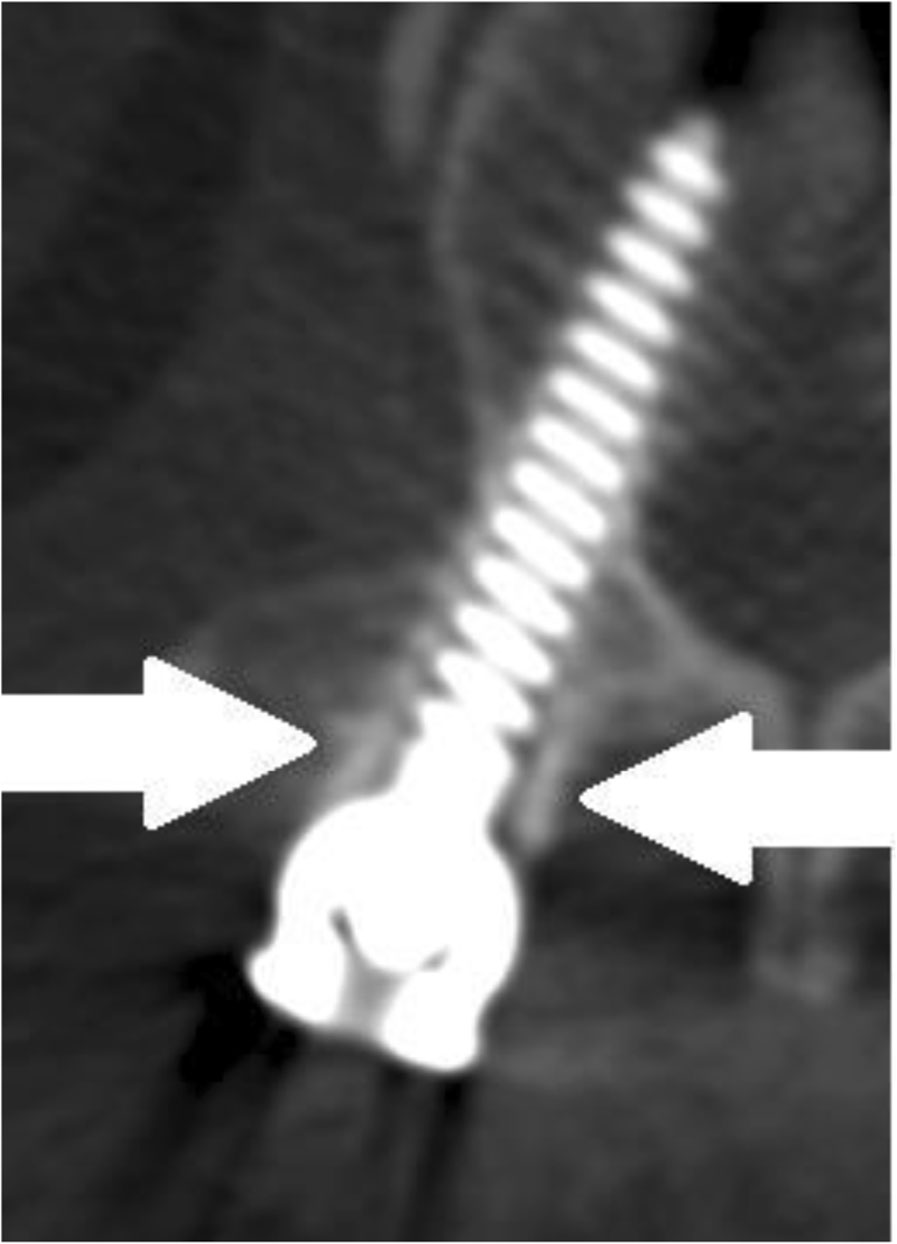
Typical CT image of metal artifact around screw tail. This picture shows metal artifact around screw tail in CT scan, which may confuse the interpretation of screw loosening
